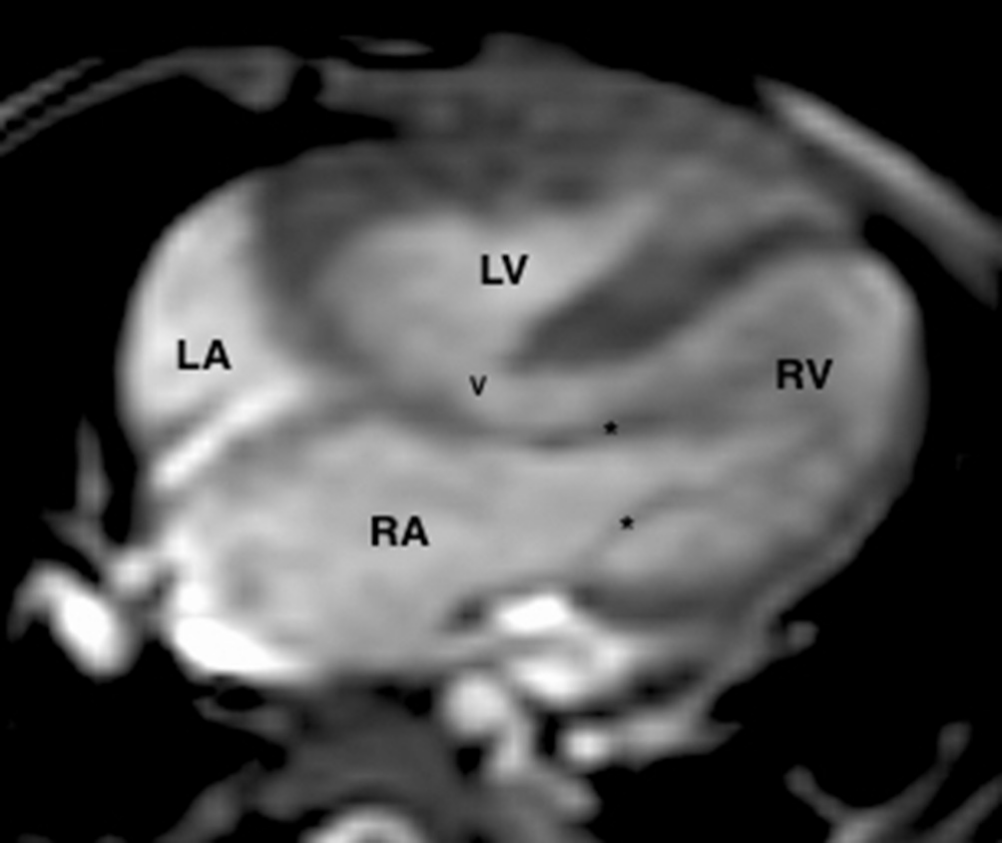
Cardiac MR image in the standard four-chamber view showing preserved RV volume (RV) and a large ventricular septal defect (v). The tricuspid leaflets (stars) are seen in proximity to the ventricular septal defect. LA, left atrium; LV, left ventricle; RA, right atrium.RV: right ventricle
